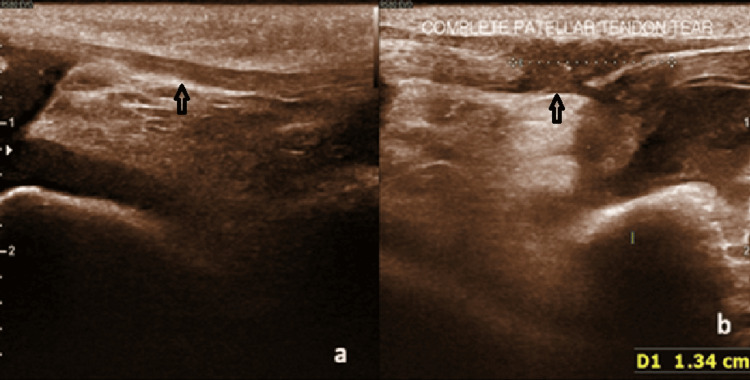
Ultrasonographic (USG) appearance of normal and torn patellar tendon. (a) USG image of a 32-year-old male showing a normal patellar tendon with preserved fibrillar echotexture and continuity. (b) USG image of a 28-year-old male demonstrating a complete patellar tendon tear with breach in tendon continuity.
